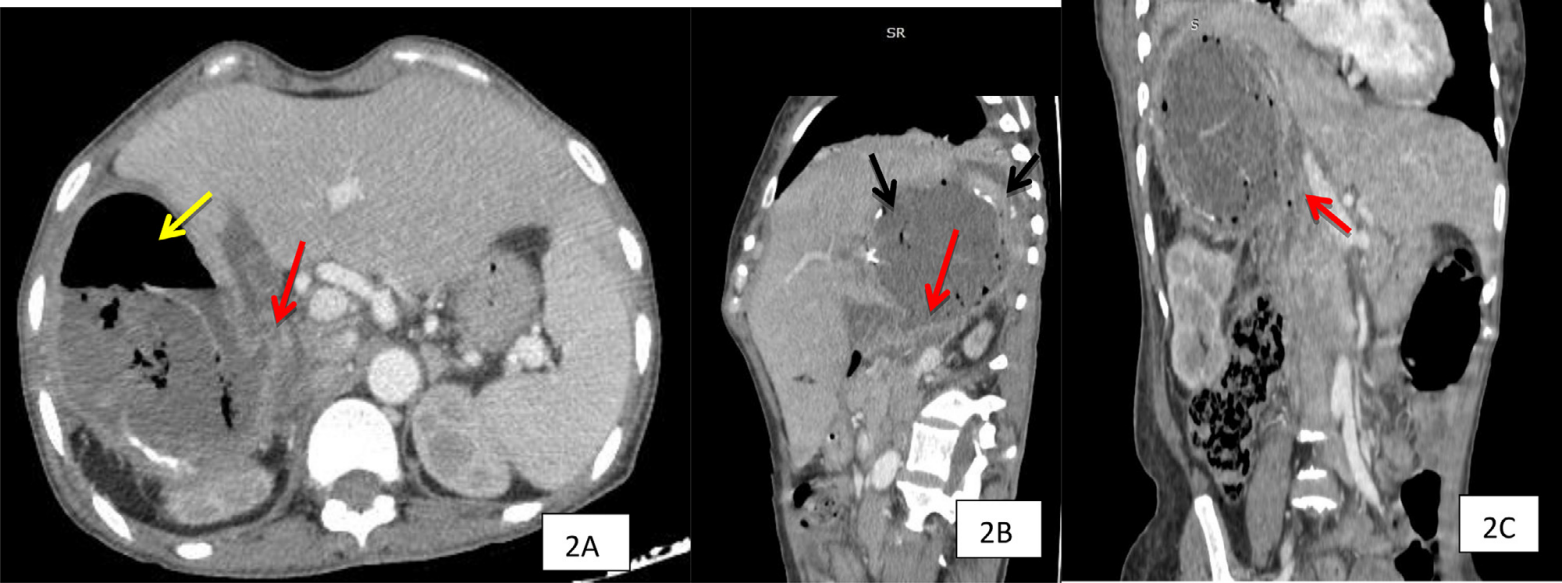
CT scan of the hepatic area with contrast in portal phase, axial (A) sagittal (B), and coronal (C) images show a hydatid cyst of the right hepatic lobe with air-fluid level (yellow arrow) and calcified walls (black arrows). They are Demonstrating also the communication between the duodenum and hepatic cyst (red arrows) (color version of figure is available online).
